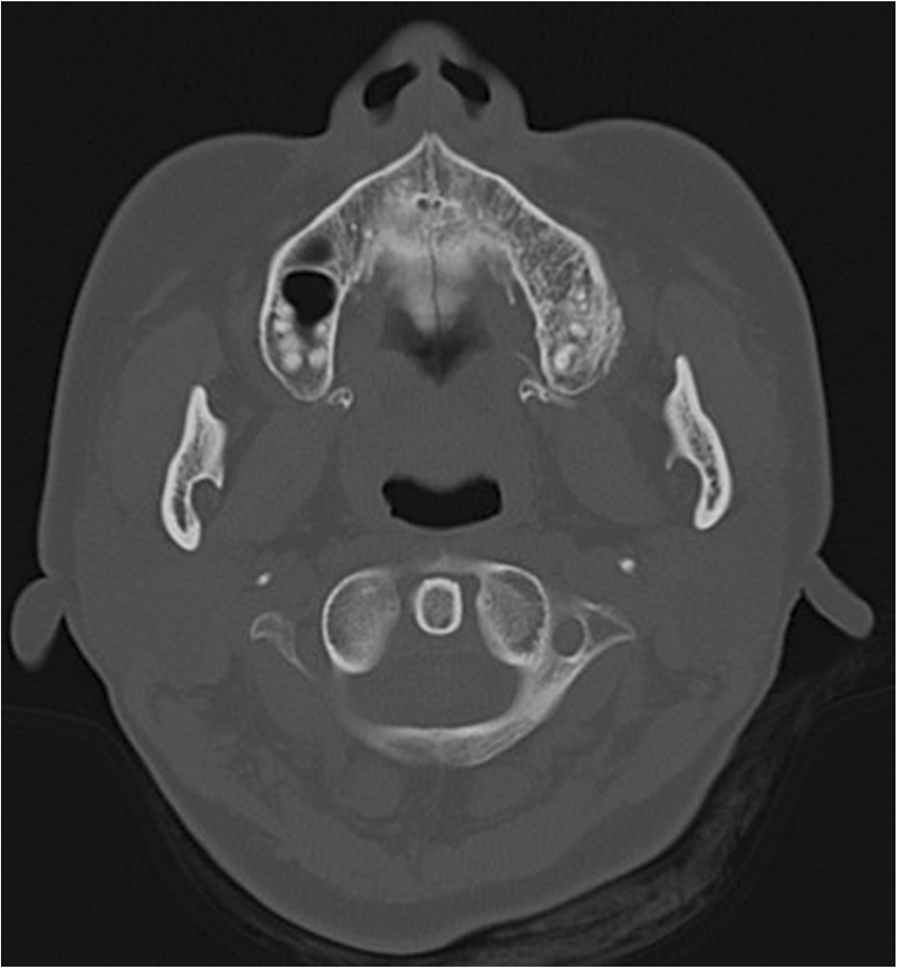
Computed tomography image at the first visit showing diffuse radiopaque lesion arising from upper left molar region
